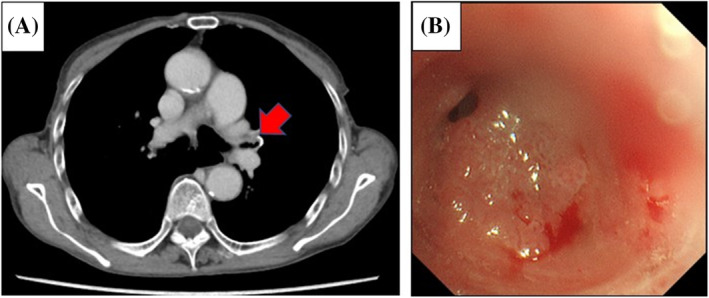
(A) Contrasted‐enhanced computed tomography (CT) revealed a 10 mm nodule in the left pulmonary hilum (arrow). (B) Bronchoscopy revealed a poor‐circumscribed tumour located at the left upper lobe bronchus.
